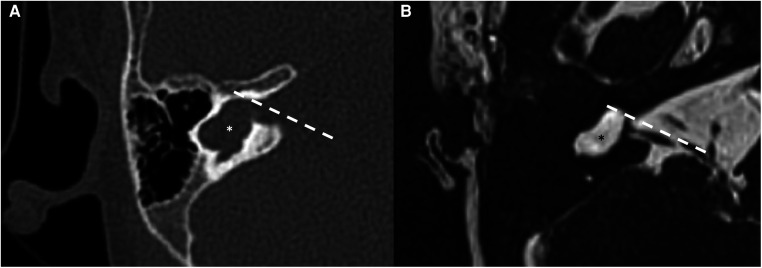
Bone-CT (A) and T2 weighted-MRI (B) imaging of a right cochlear aplasia. Note the complete development of the cystic cavity behind the axis of the internal auditory canal (dashed white line). The posterior labyrinth is cystic and it also represents the semicircular canals (asterisks).
